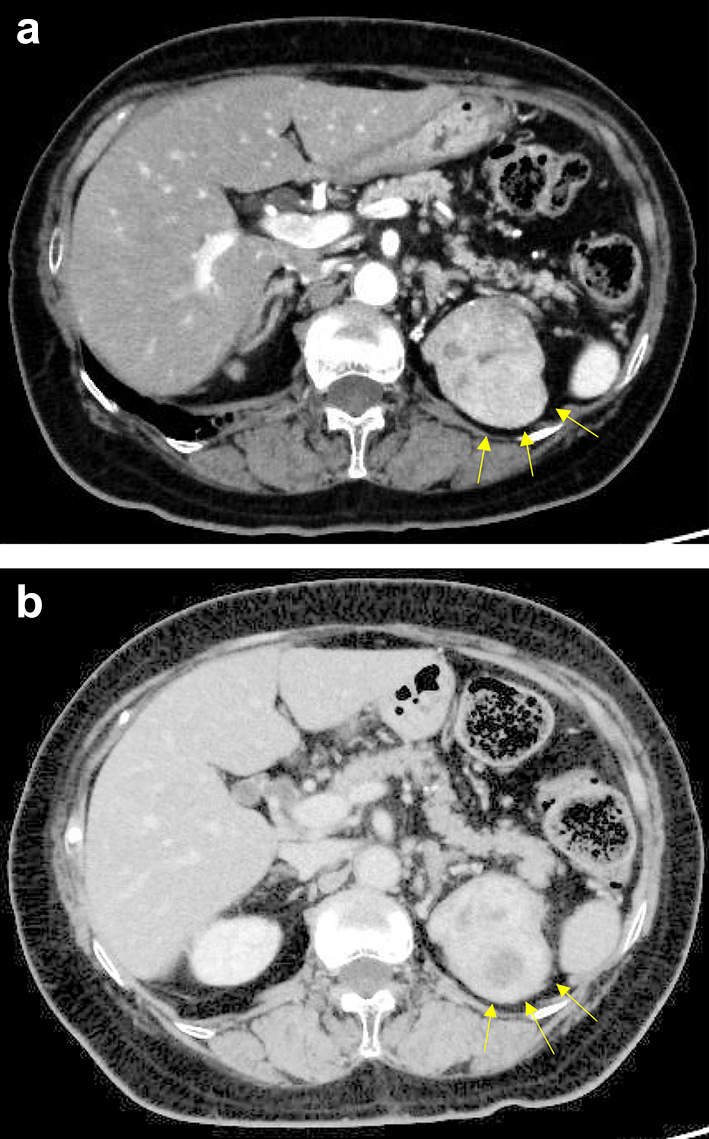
Contrast-enhanced computed tomography image showing a tumor with internal heterogeneous contrast accumulation in the left kidney. a Before and b after intratumoral metastasis of sigmoid colon cancer to renal cell carcinoma
